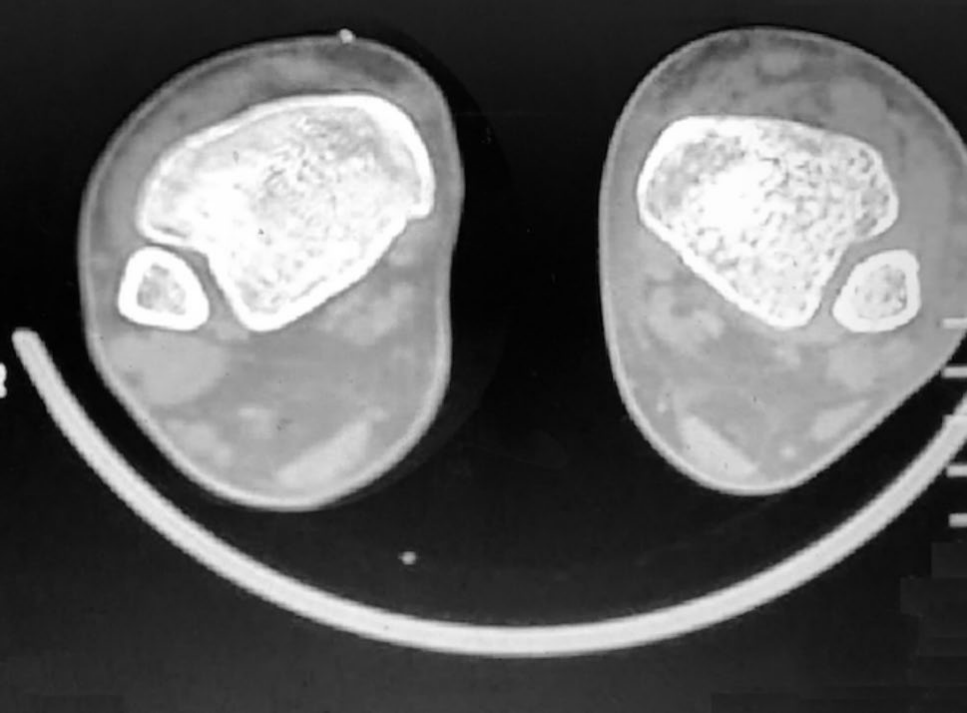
CT 1.5 cm above ankle at both sides at 3 months after surgery. The right side is the operated ankle
